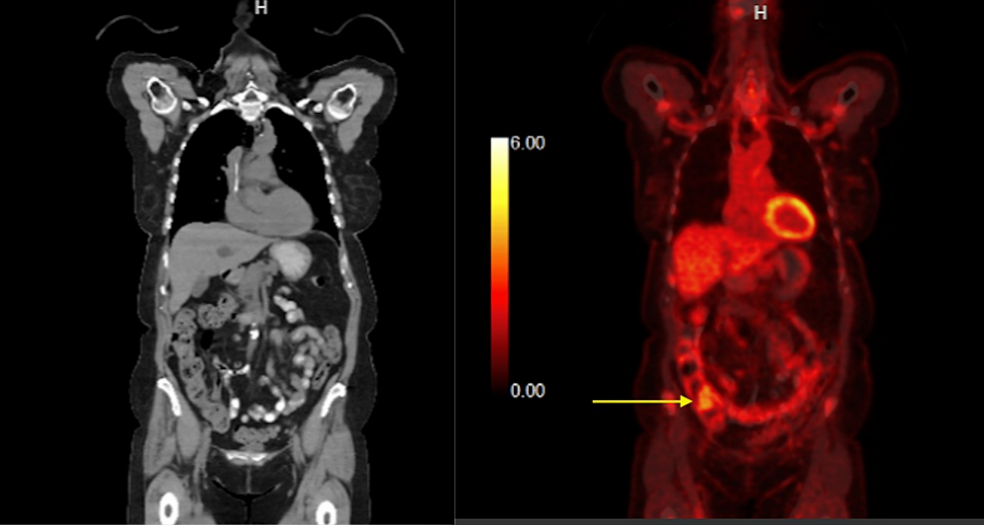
CT and PET scans reveal a significant reduction in size and minimal uptake on PET scan by cecal mass compared to pre-chemotherapy, indicating a positive response to chemotherapy (R-CHOP). PET: positron emission tomography; R-CHOP: rituximab, cyclophosphamide, hydroxydaunorubicin, vincristine, and prednisone.
